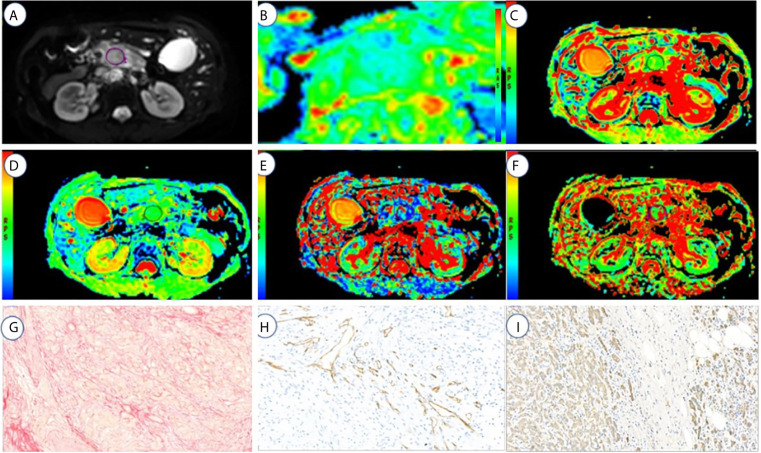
A 72-year-old male with high fibrosis PDAC. (A–F) represents the DWI, ADC(0,500), ADC(0,800), D, f, and D* values map, respectively. (G–I) represents the fibrosis, microvascular density, and tumor cell dyeing map, respectively. ROI was set in PDAC on DWI, ADC _(0, 500)_, ADC _(0, 800)_, D, f, and D* values maps. The cancer tissue showed different signals from normal tissues in the DWI, ADC _(0,500)_ (1.7 μm^2^/ms), ADC _(0,800)_ (1.6 μm^2^/ms), D (1.53 μm^2^/ms), f (13.05%), and D* (125.3 μm^2^/ms). Fibrosis, MVD, and tumor cell are 21, 6.5, and 29%, respectively.
